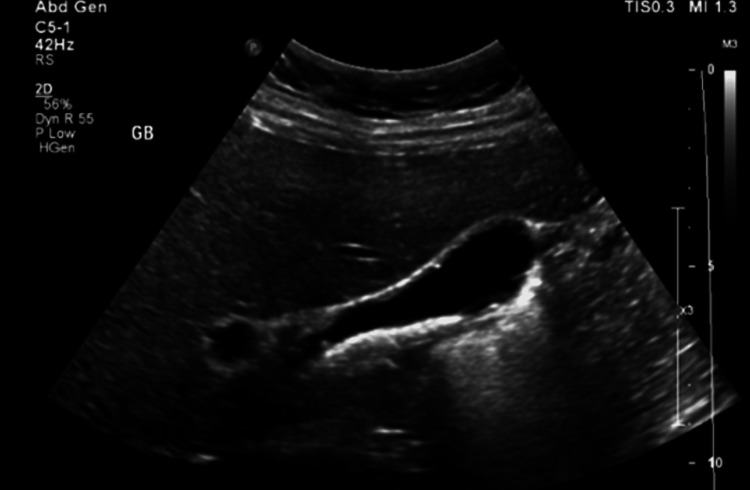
Ultrasonography of the gall bladder showing multiple gallstones.
